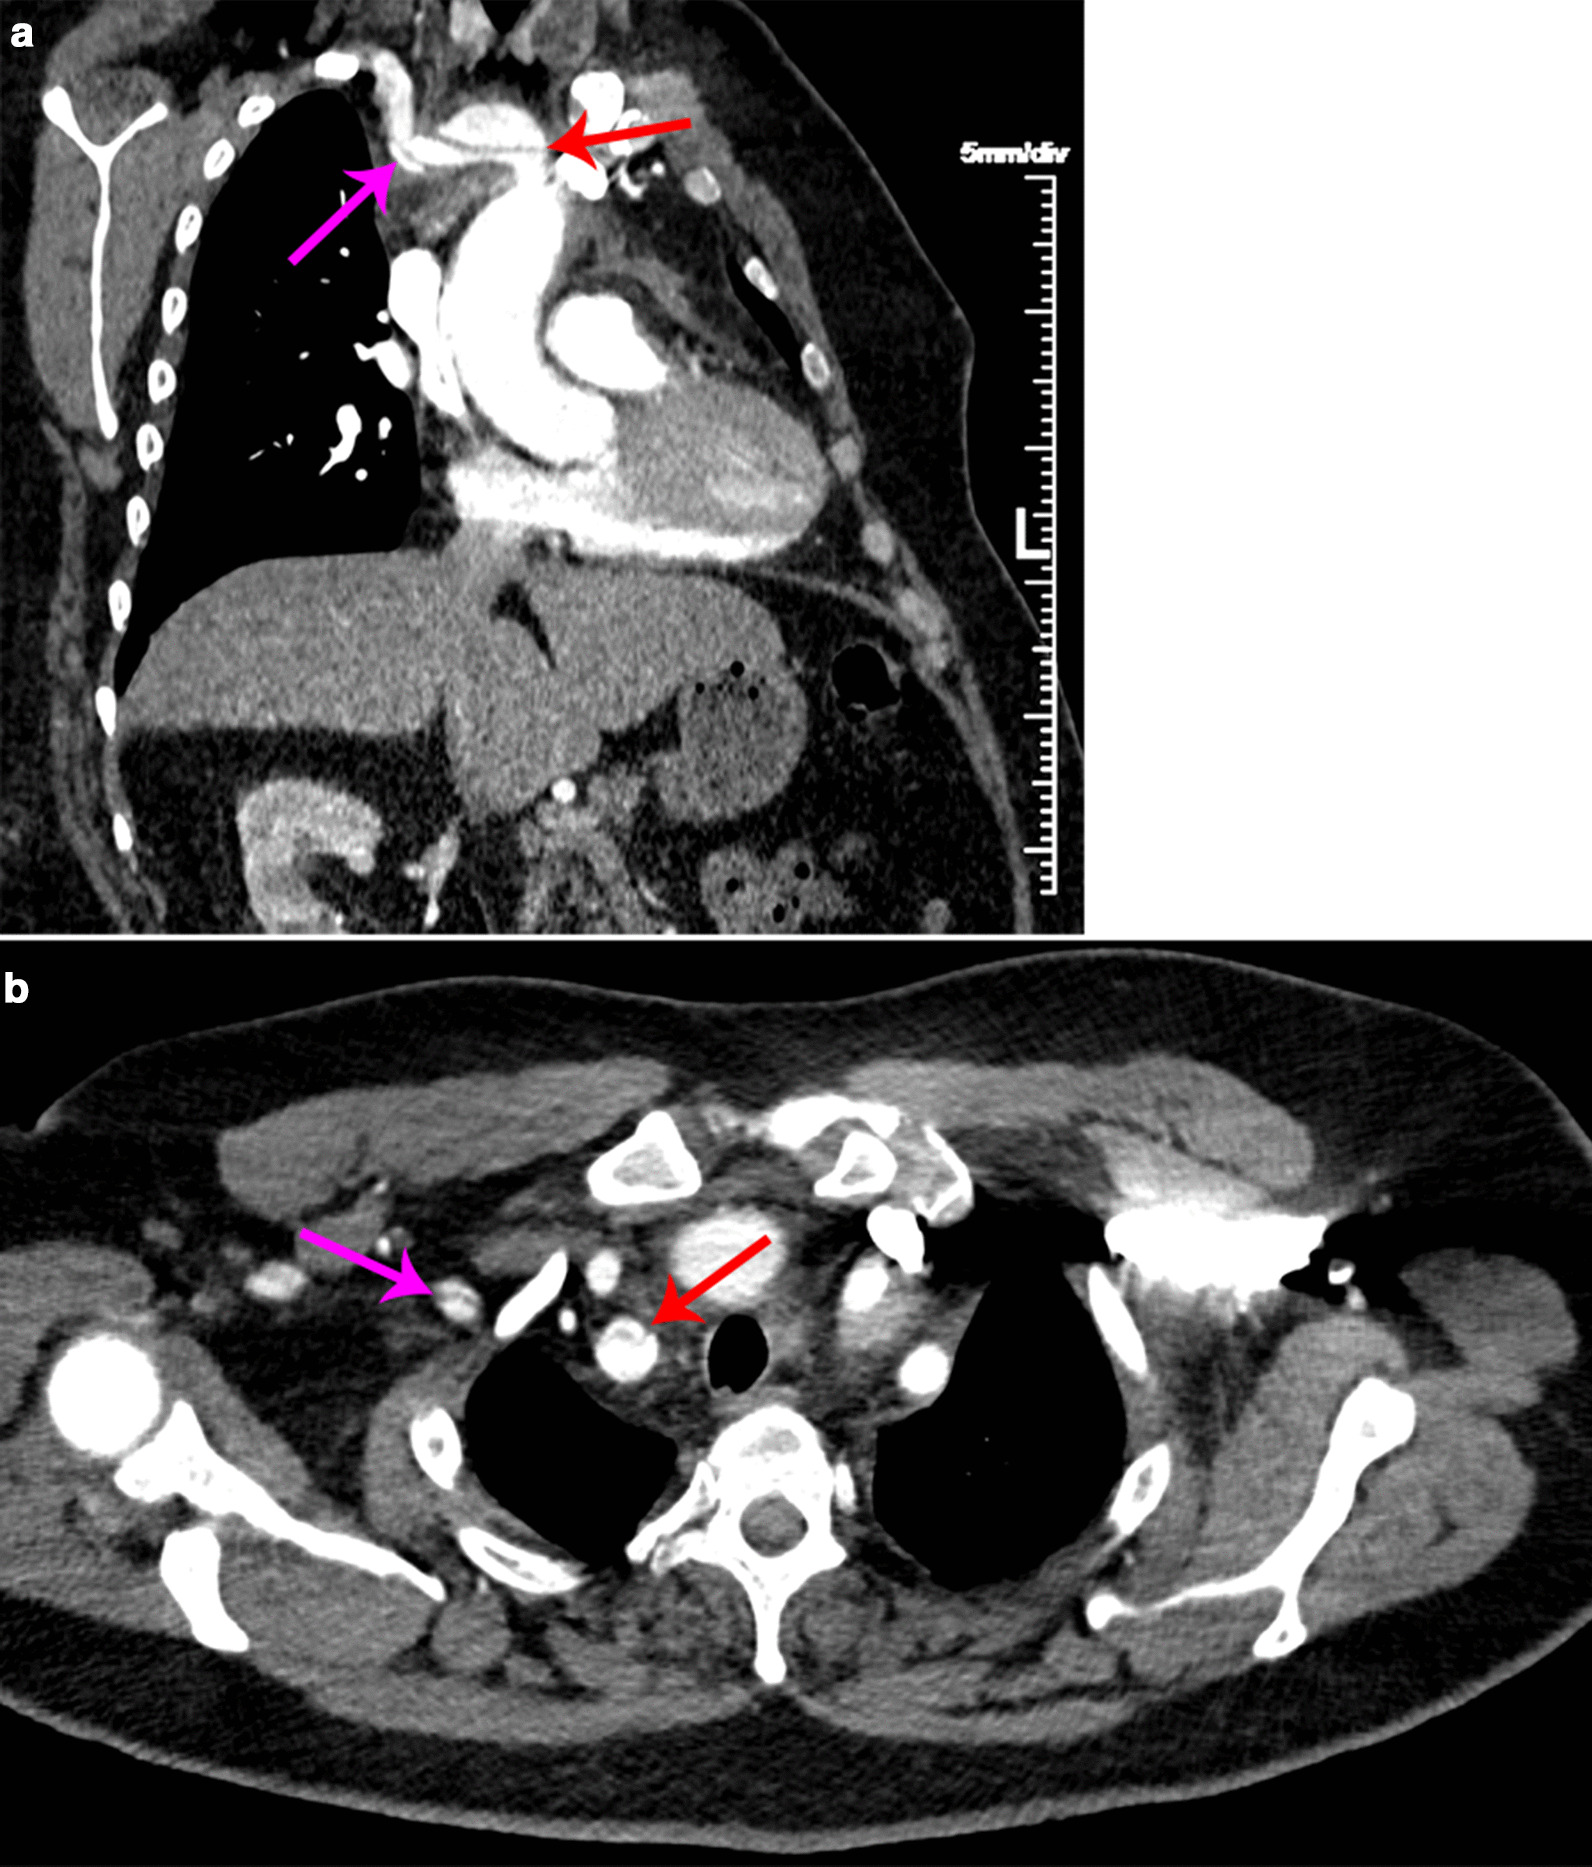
Computed tomographic angiography chest results at initial admission. Innominate artery demonstrates distinct widening on coronal oblique view (a) after takeoff from the aortic arch. This (red arrow, a) represents the origin of dissection, which separates the true lumen (below red arrow, a) from the false lumen (above red arrow, a). Extension distally into the subclavian artery is observed (pink arrow, a). Axial imaging (b) shows true and false lumens in both the subclavian artery (red arrow, b) and the axillary artery (pink arrow, b), evidencing distal extension of dissection. Aortic involvement is absent—no true and false lumens are identified.
